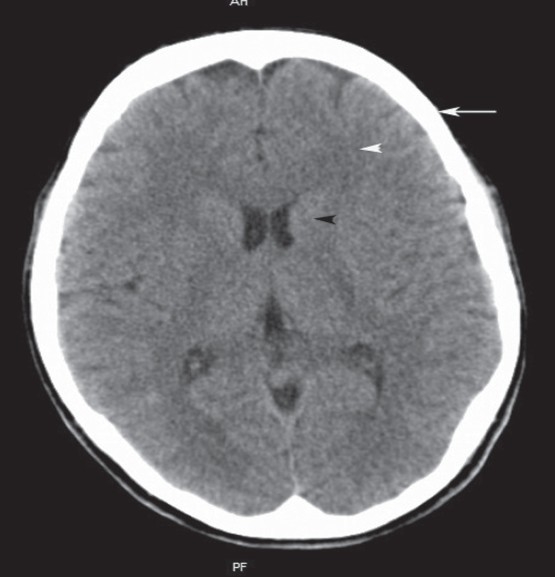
An image from CT of head showing gray matter (black arrowhead) denser than white matter (white arrowhead). Tissues with high calcium content like bone are very dense and appear white (arrow)
